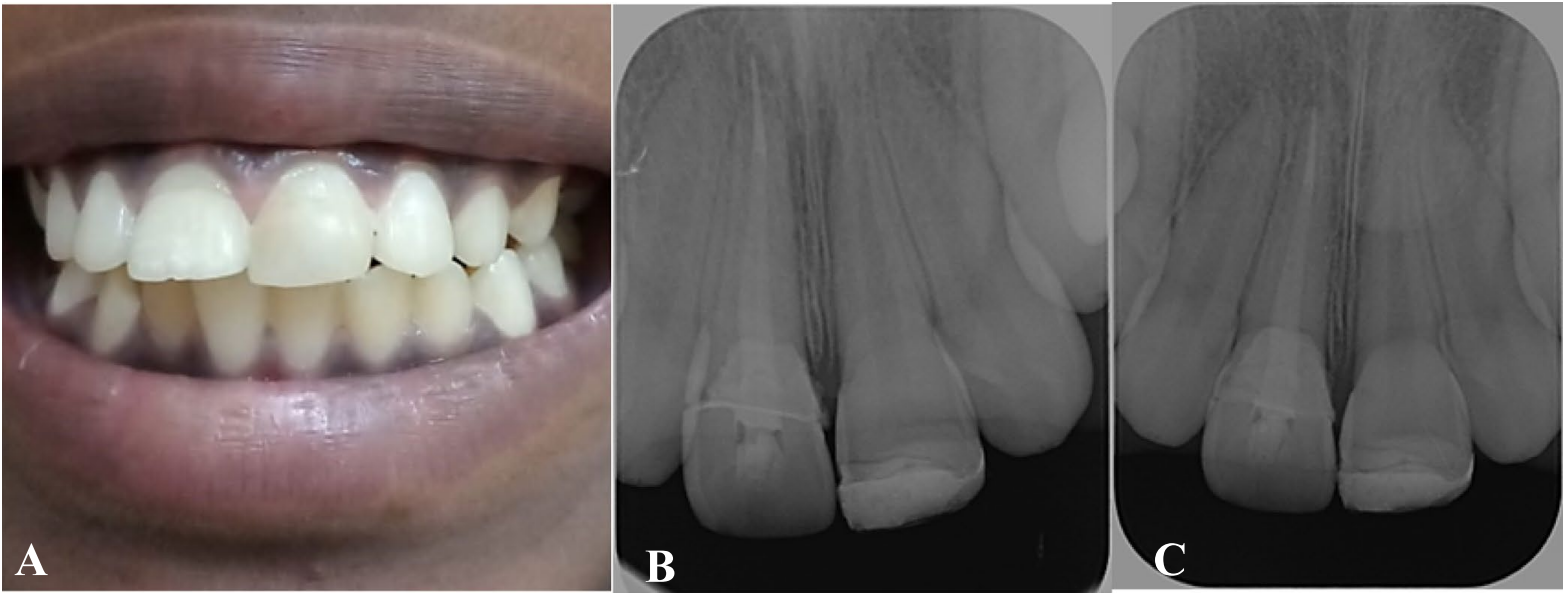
Post‐operative clinical photos and radiograph. (A) Frontal view after 12 months. (B) Periapical radiograph after 6 weeks. (C) Periapical radiograph after 12 months.
